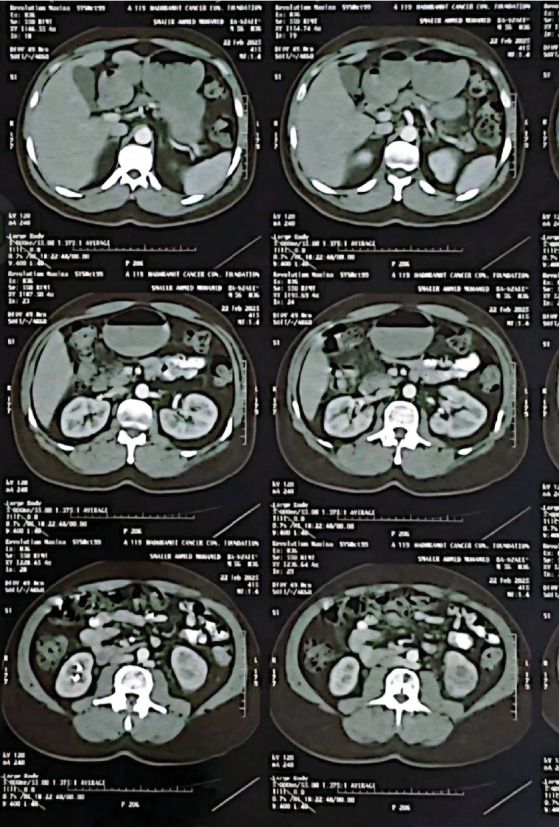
CT abdomen show left malignant lower pole renal mass.
